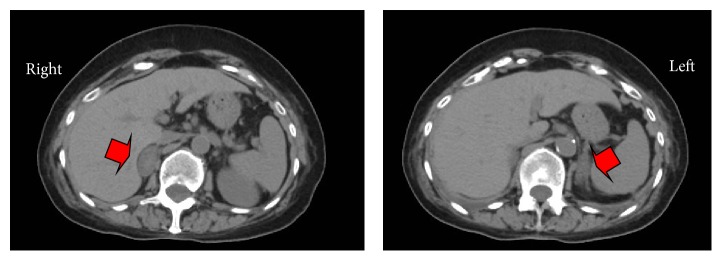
Abdomen CT showing bilateral adrenal enlargement, highlighted by red arrows, with a hyperdense central area of 2 cm of maximum diameter (HU 25–35) in right adrenal gland.
